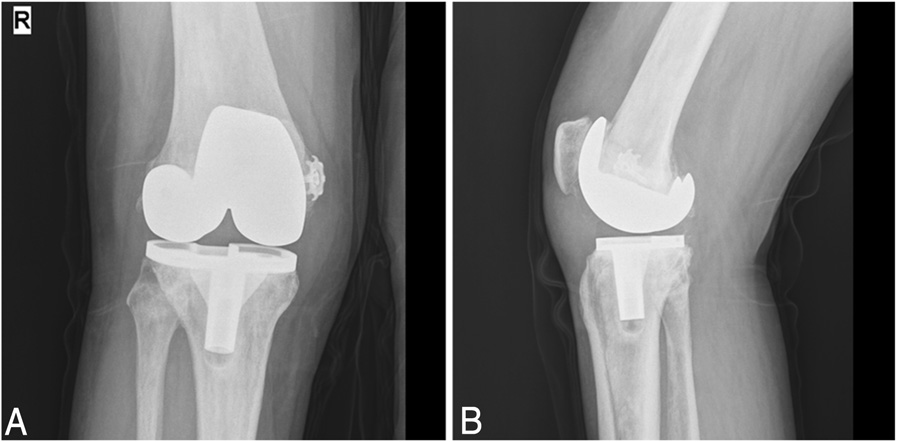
One-year postoperative radiograph of the right knee. Antero-posterior (a) and lateral (b) views show satisfactory position of the components
